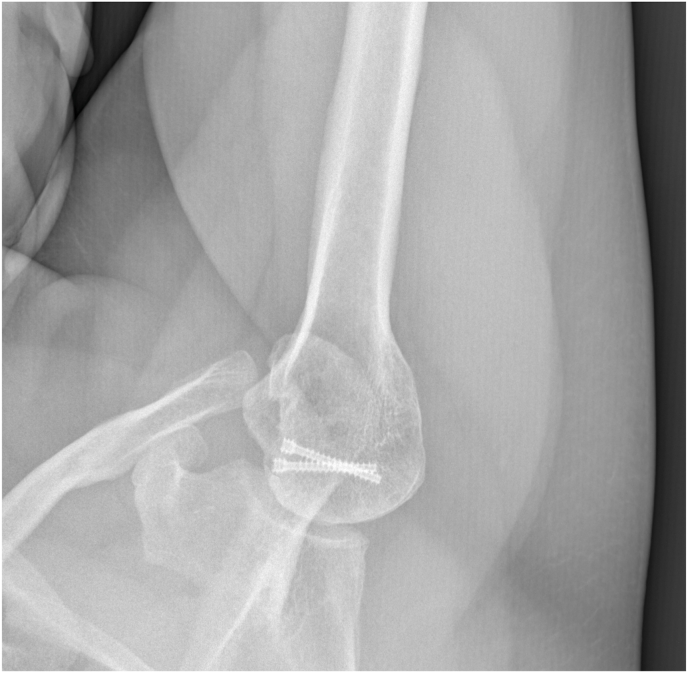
Axial X-ray of the left shoulder in a 28-year-old woman after the modified McLaughlin procedure, fixation with 2 cannulated screws.
